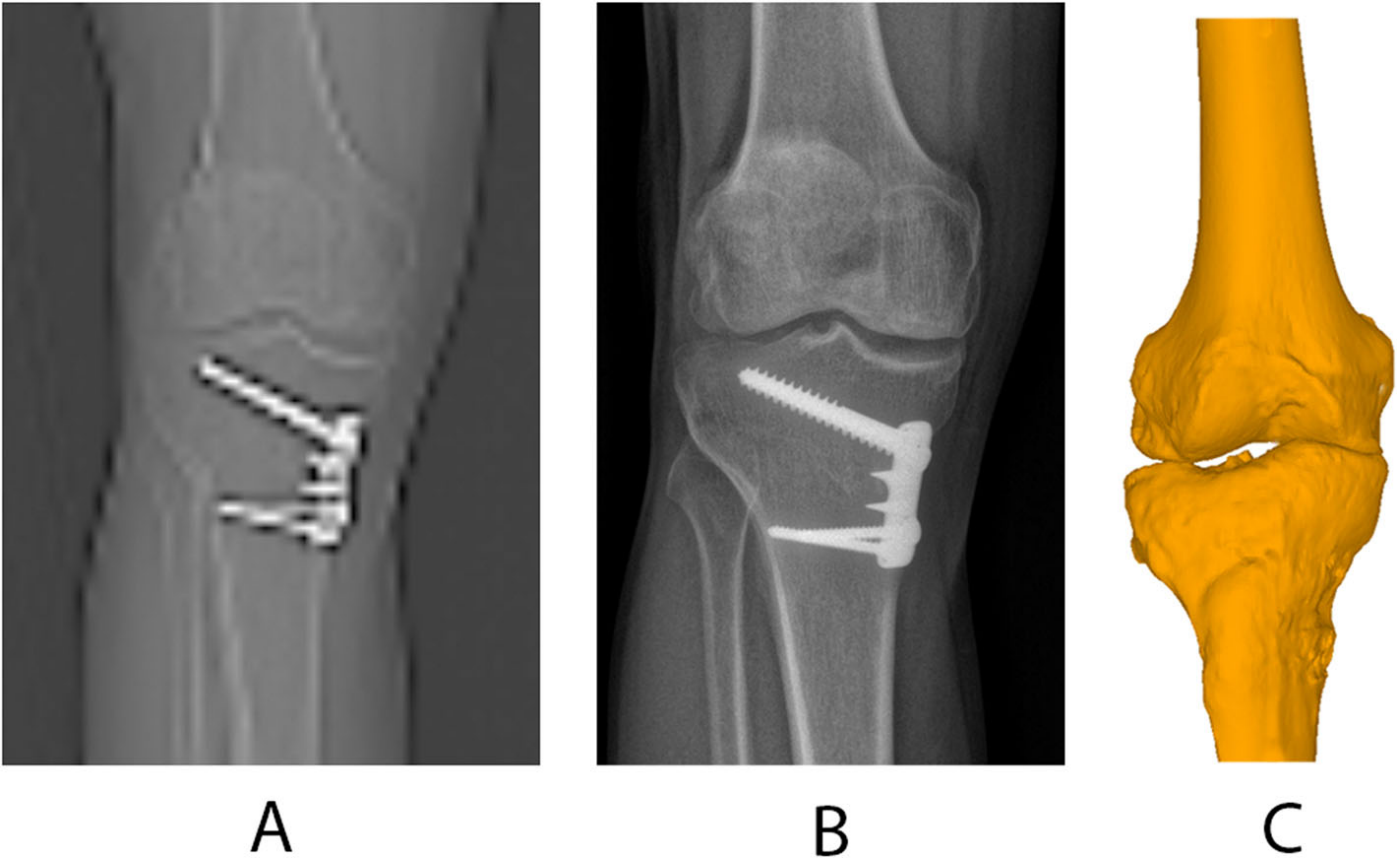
Substantial differences in the depiction of the tibial plateau between 2D and 3D. While the 3D model c can accurately represent the patients’ anatomy, the 2D projections (scanogram a and radiograph b) do not adequately display the full information
